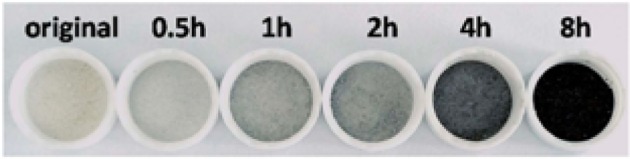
The powders obtained after different ultrasound irradiation duration. Reprinted with permission from [89]. Copyright (2015) Springer Nature
